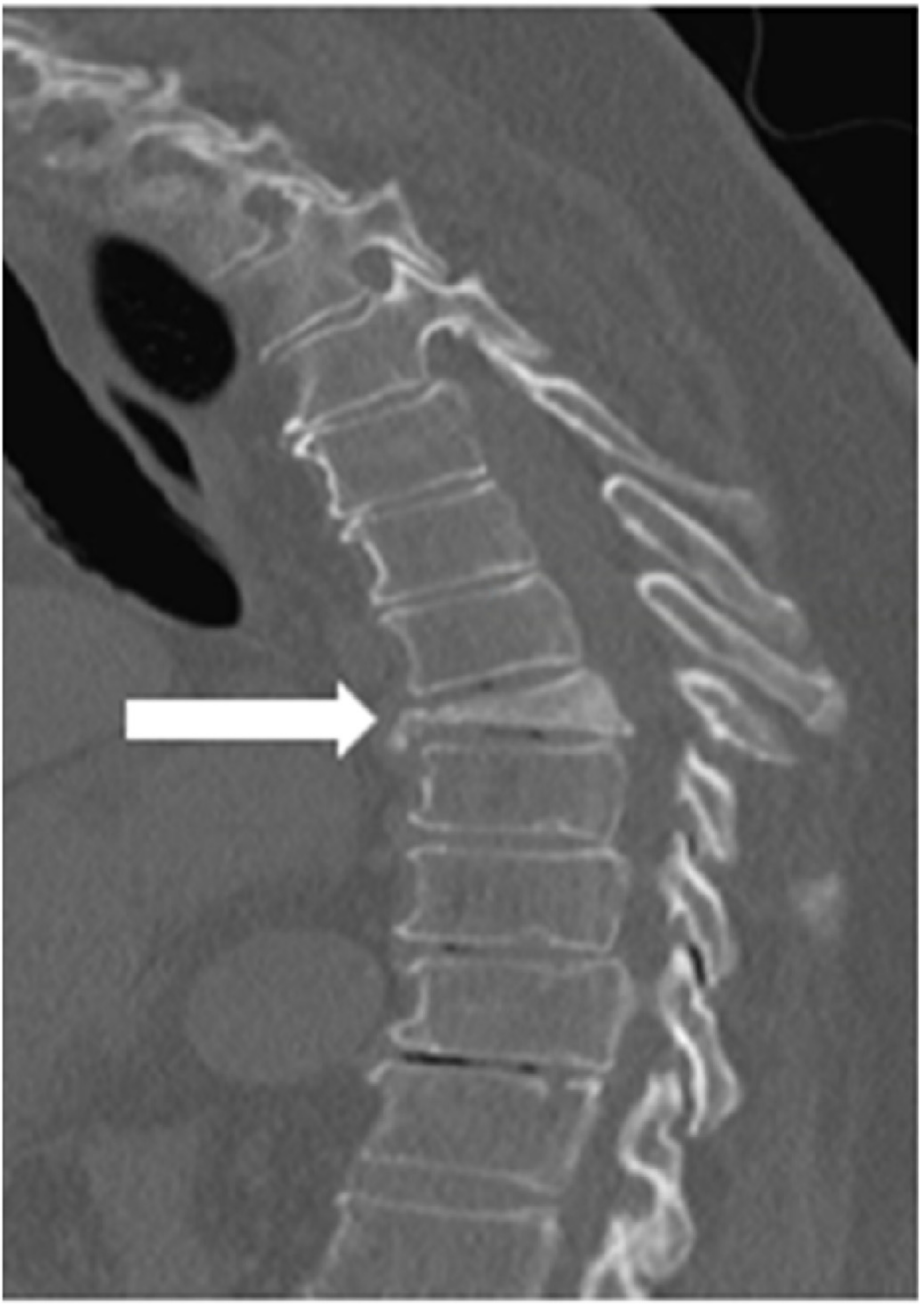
Sagittal CT reveals T8 compression fracture with 75% height loss.
